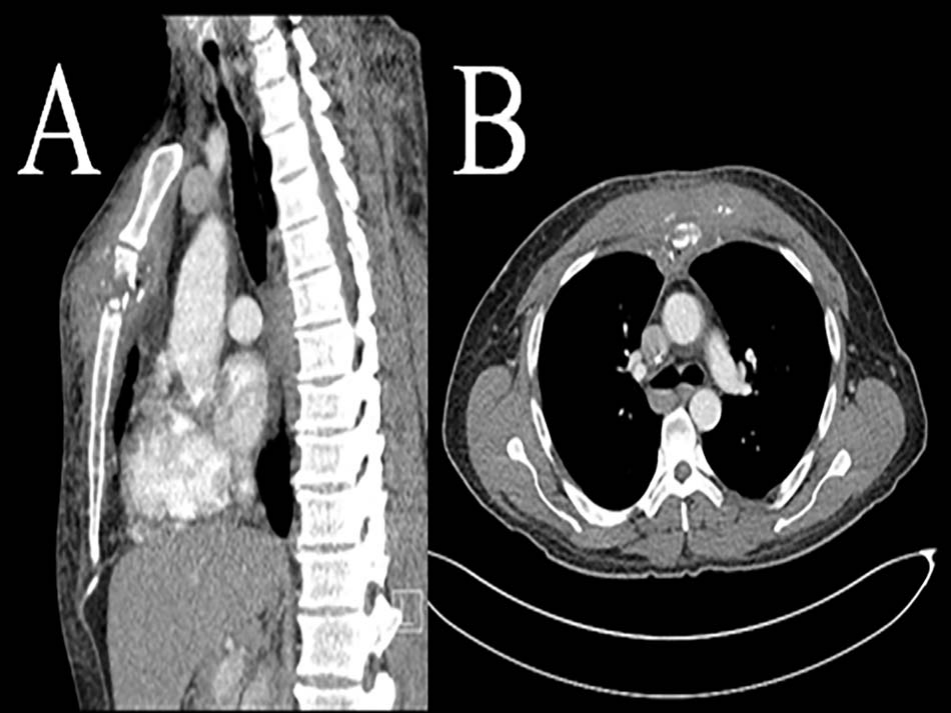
Computed tomography (CT scan) sternomanubrial joint bone erosion and phlegmon. Computed tomography (CT scan): A (sagittal view) and B (axial view).
